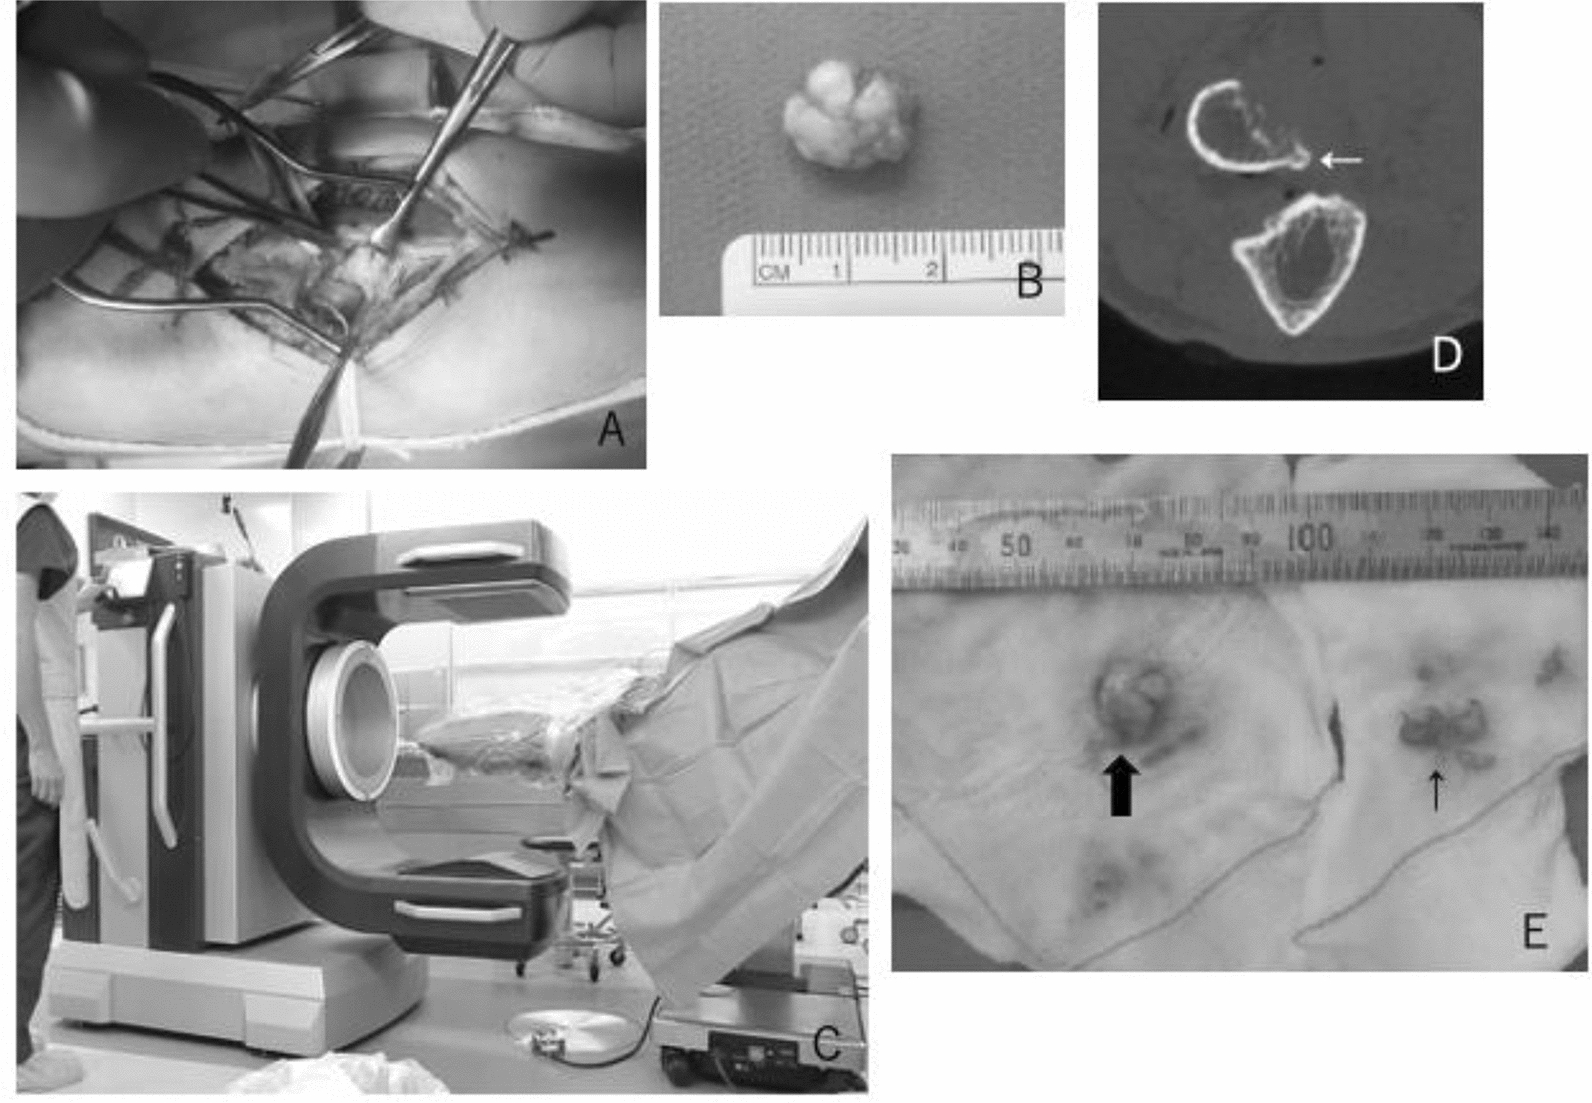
A Intraoperative picture of the left elbow showed the abnormal prominence of the bicipital tuberosity of the radius. B 11 mm × 15 mm of the lesion was removed. C Intraoperative CT scan was performed using 3D Accuitomo M (Morita Co. Ltd., Kyoto, Japan). D Intraoperative computed tomography of the right elbow showed the remaining spur of the bicipital tuberosity of the radius (arrow). E Additional resection was performed (small arrow). The large arrow shows the resected part before the computed tomography (already shown in B)
